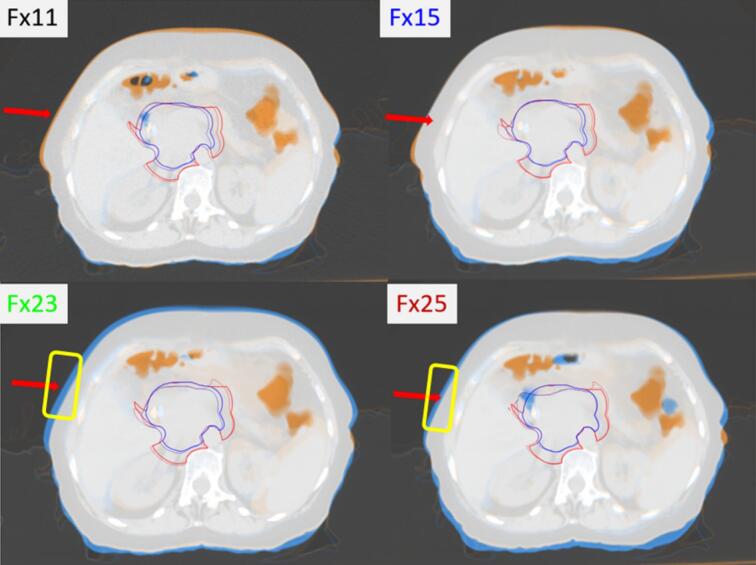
Difference between planning CT (sky blue) and control CTs (orange) for each monitored fraction. The patient's weight loss was visible towards the end of treatment. High-risk and low-risk CTV are shown in dark blue and red, respectively. The direction of the monitored beam is indicated by the red arrow. Yellow boxes highlight the area of the patient surface shift. (For interpretation of the references to colour in this figure legend, the reader is referred to the web version of this article.)
